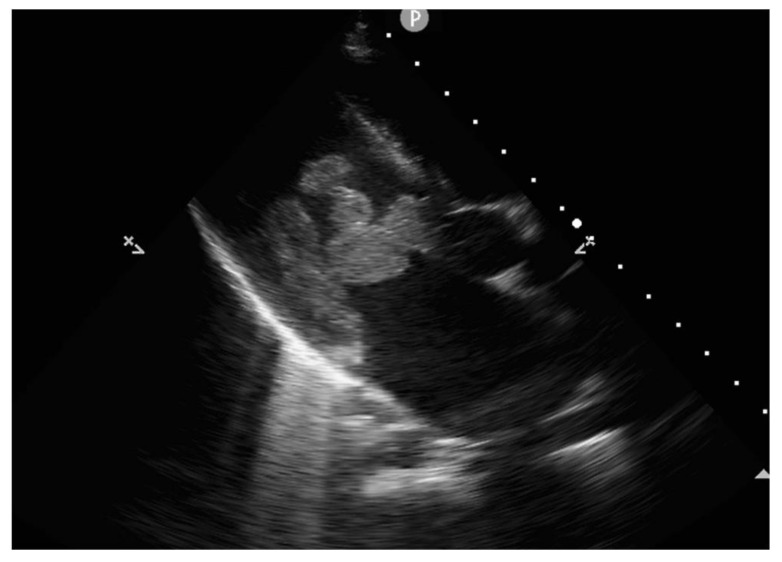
Echocardiography shows numerous vegetations at the mitral level, of different shapes and dimensions, obstructing the left ventricular inflow, with high mobility especially of the most distal one.
